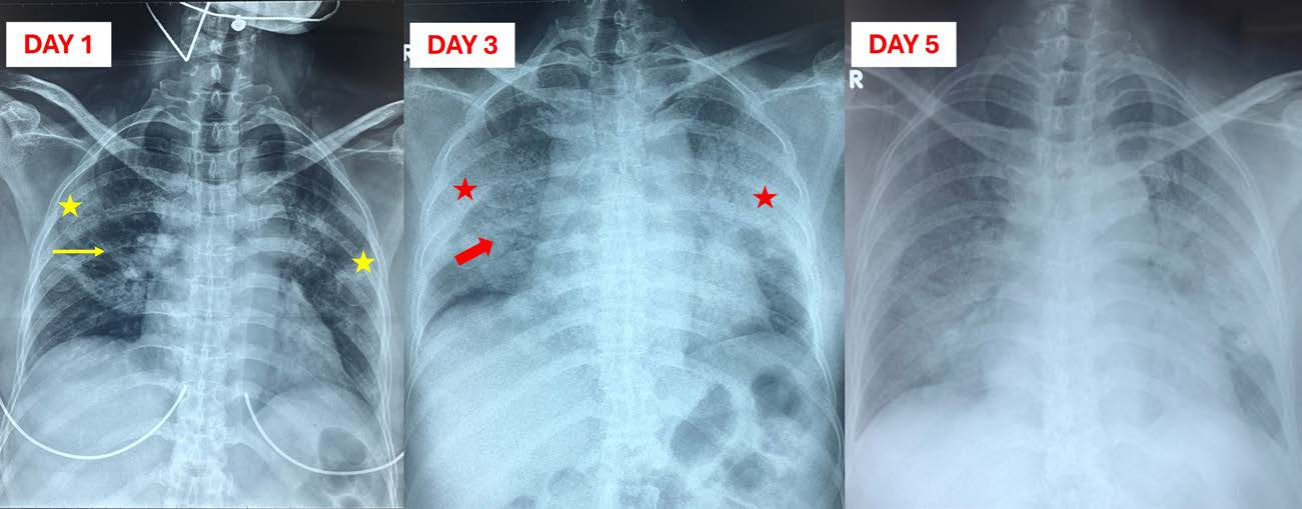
Chest X-ray from day 1 to day 5 postadmission. Day 1: Chest X-ray shows linear interstitial patterns (yellow line arrow) and focal consolidations (yellow star). Day 3: Lesions have progressed to bilateral lung consolidations (red arrow) with air-bronchogram signs (red arrow). Day 5: Consolidations observed on day 3 have further worsened, indicating a severe progression of the lung damage.
